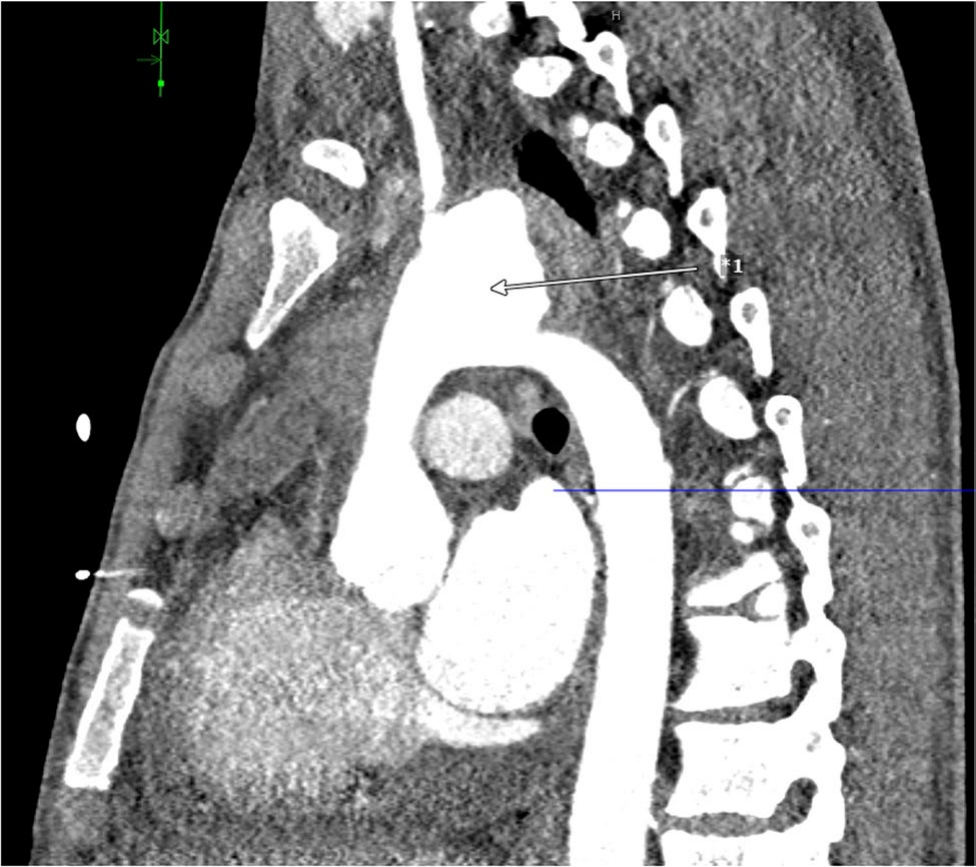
CT scan of the Thorax at the day of operation (sagittal plane), *1 pseudoaneurysm
